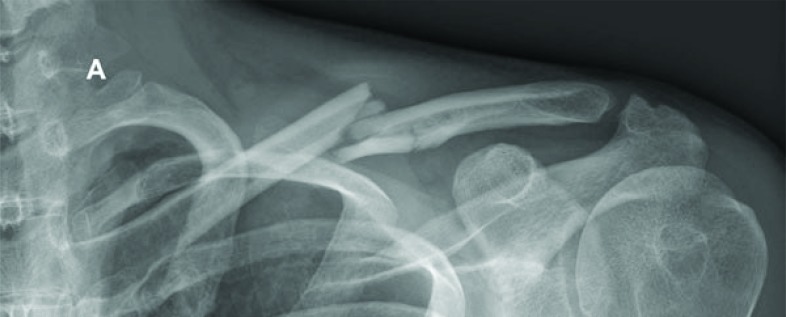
The antero-posterior radiograph shows an acute multifragmentary midshaft fracture of the clavicle with slight displacement and preserved contact of the bone fragments.
